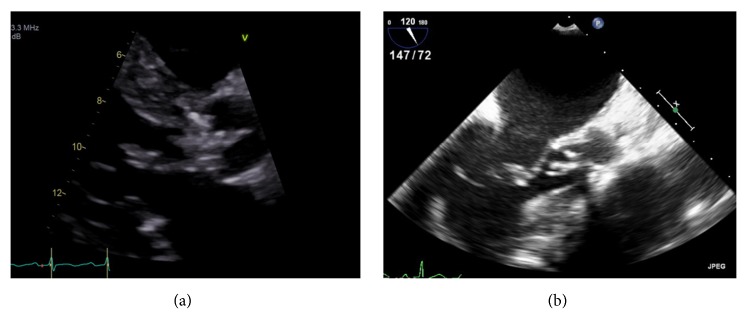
(a) Transthoracic echocardiogram on parasternal long axis view shows independently mobile echo dense structure of 1.61 cm x 0.82 cm on the LVOT side, likely vegetation. (b) Transesophageal echocardiogram mid esophagus long axis view shows the aortic valve with the aortic valve vegetation.
